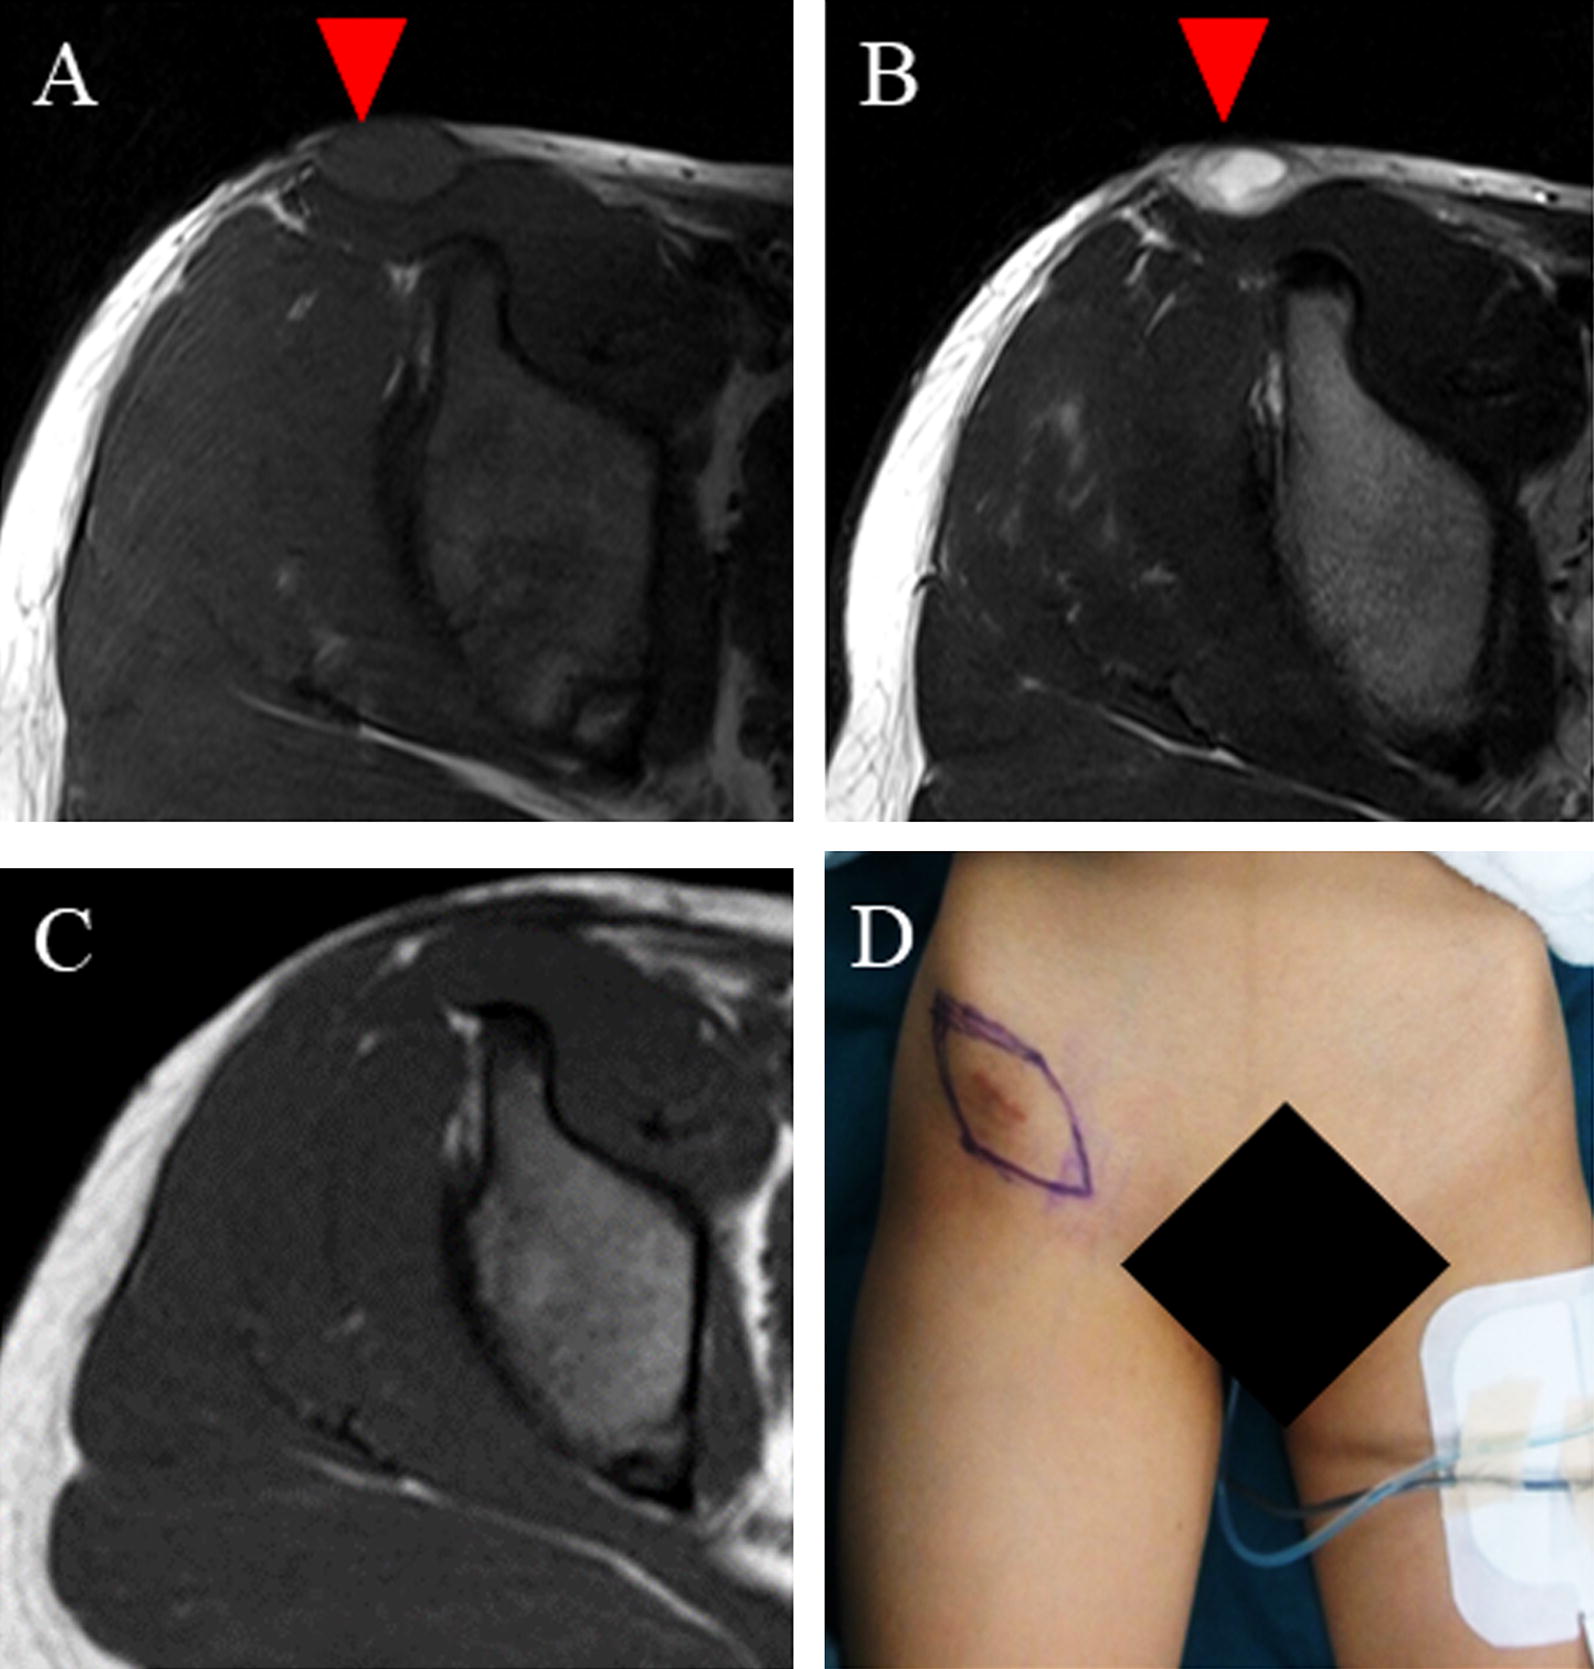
A Coronal image of the iliac region acquired using T1-weighted magnetic resonance imaging (MRI). B Coronal image of the iliac region acquired using T2-weighted MRI. C Coronal image of the iliac region acquired using T2-weighted MRI after unplanned resection. Red arrowheads indicate the tumor. D Visual appearance of the iliac region
